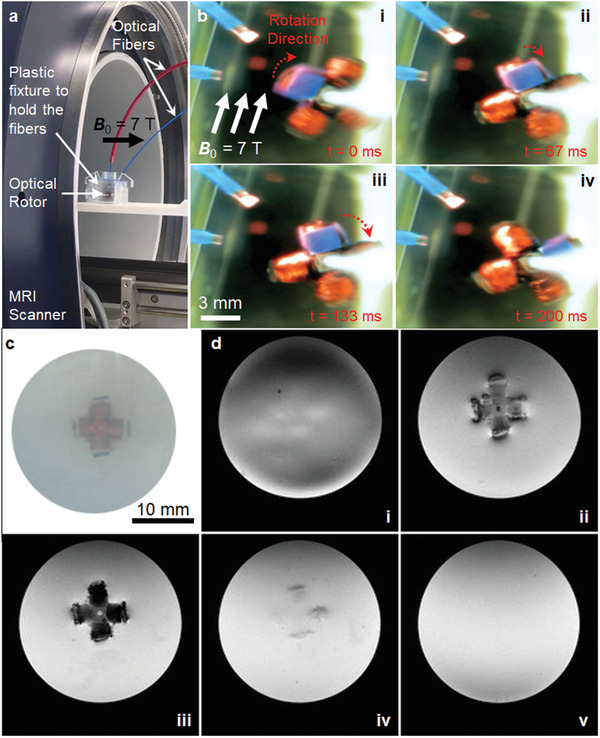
Test results of the four‐module rotary actuator inside the MRI scanner. a) Insertion of the rotor into the preclinical MRI scanner (*B*_0_ = 7 T) with the plastic holders and fiber optic cables. b) Representative time‐lapses of the rotor motion inside the MRI scanner with optical power applied through the fibers. c) Optical image of the rotor inside the agar gel. d) FLASH coronal MR image slices of the same rotor inside the MRI scanner showing no image artifacts when it is not operated (off).
